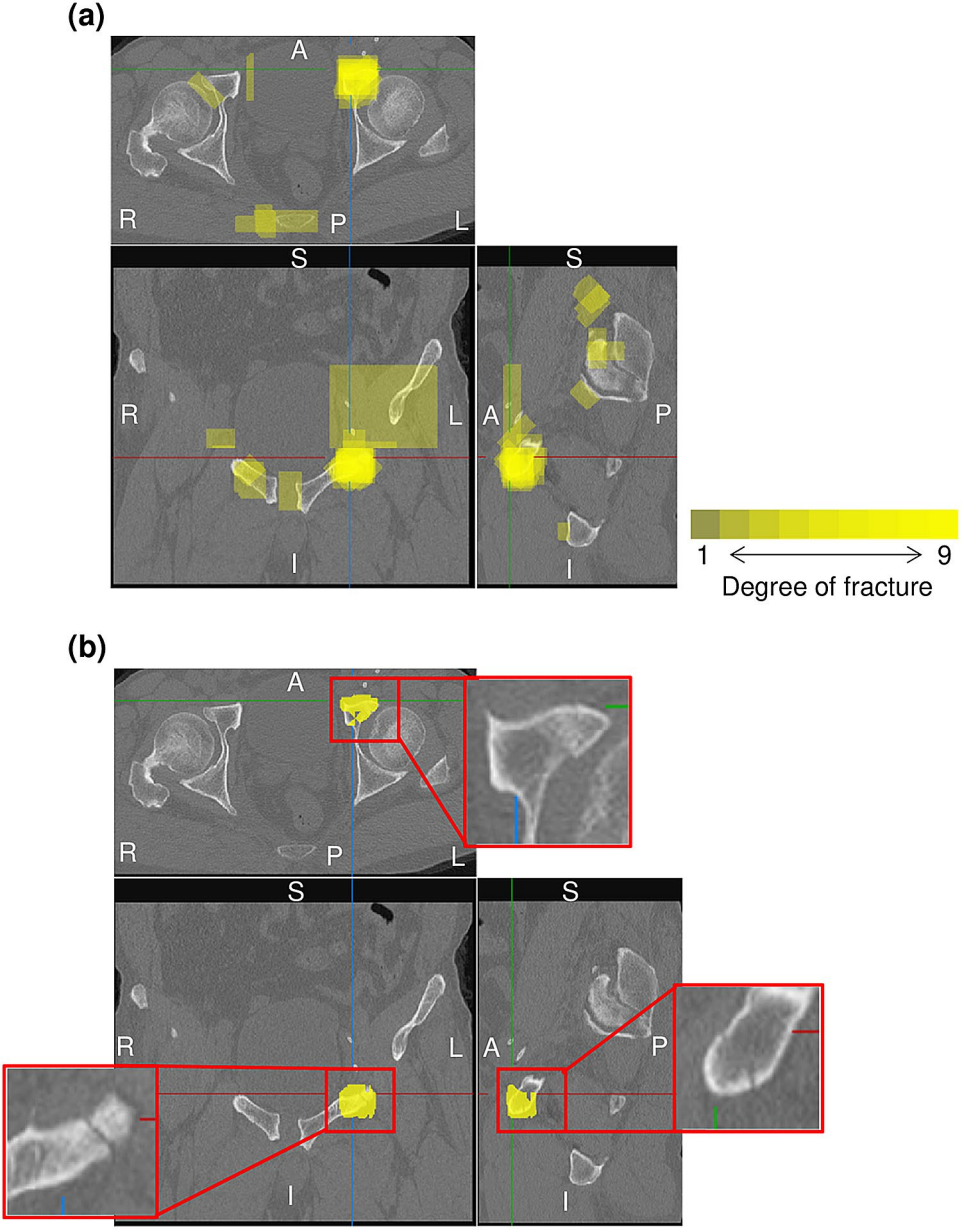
Estimated degree of fracture on multiplanar reconstruction images. Top: axial image; bottom-left: coronal image; bottom-right: sagittal image. L: left; R: right; A: anterior; P: posterior; S: superior; I: inferior. (a) Integrated 3D fracture candidate region overlapping on CT images ($${C}_{th}$$: 0.2). Yellow represents the degree of fracture. (b) Resultant 3D fracture region ($${I}_{th}$$: 6). Yellow represents the detected region. The enlarged image shows raw CT images for the detected fracture region.
